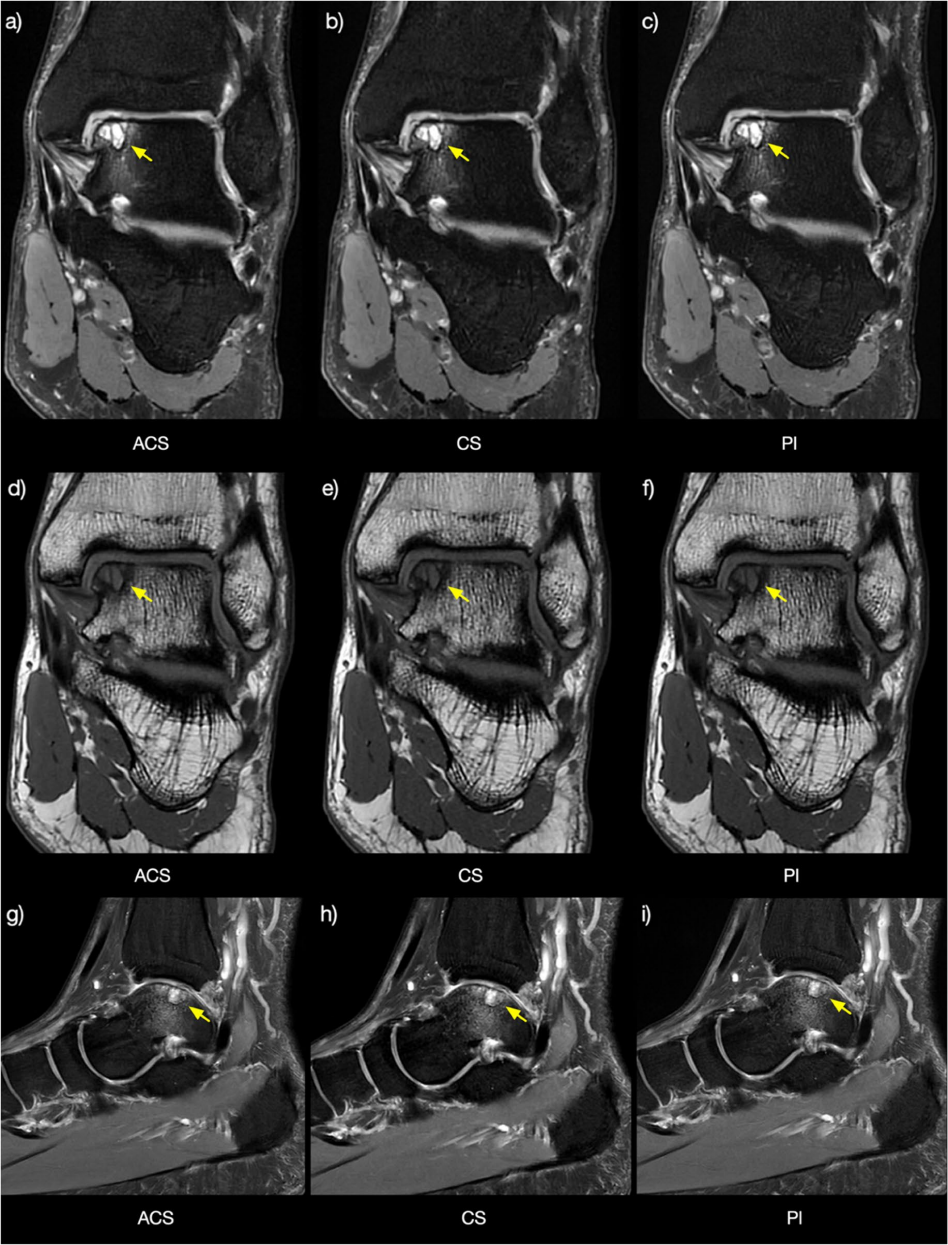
Coronal PD-weighted fat-sat and T1-weighted images acquired using ACS (a, d), CS (b, e), and PI (c, f) showing the ankle of a 34-year-old male patient with an osteochondral lesion (arrows, grade 4). Sagittal PD-weighted fat-sat images acquired with ACS (g), CS (h), and PI (i) showing bone marrow changes adjacent to the osteochondral lesion (arrows). *ACS* Artificial intelligence-assisted compressed sensing, *CS* Compressed sensing, *PD* Proton density, *PI* Parallel imaging
